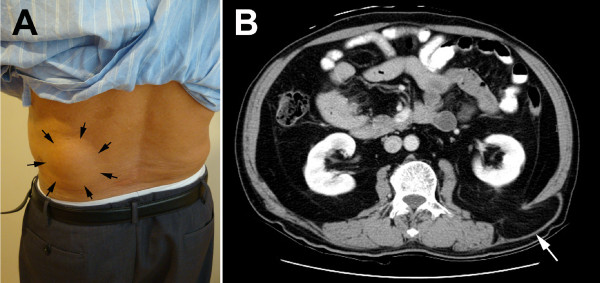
Physical examination and computed tomography findings on admission. (A) Left lower back bulging mass of size 10cm × 6cm (arrows). (B) Abdominal computed tomography demonstrating retroperitoneal fat protruding through the superior lumbar triangle (arrow).
